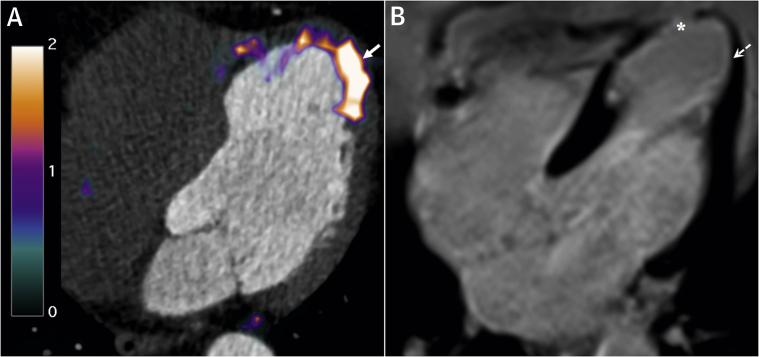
Post-Infarction Myocardial Inflammation Identified by ^68^Ga-DOTATATE PET (A)^68^Ga-DOTATATE positron emission tomography (PET)-computed tomography image (scale bar: standardized uptake values) demonstrating residual inflammation (arrow) in (B) partially viable myocardium with subendocardial infarct (dashed arrow), bordering full-thickness scarring (asterisk) confirmed by late gadolinium enhancement magnetic resonance imaging, 4 years after a left anterior descending artery myocardial infarction. ^18^F-FDG positron emission tomography imaging reproduced a near-identical pattern of abnormal myocardial tracer uptake. Stress magnetic resonance imaging was negative for ischemia.
